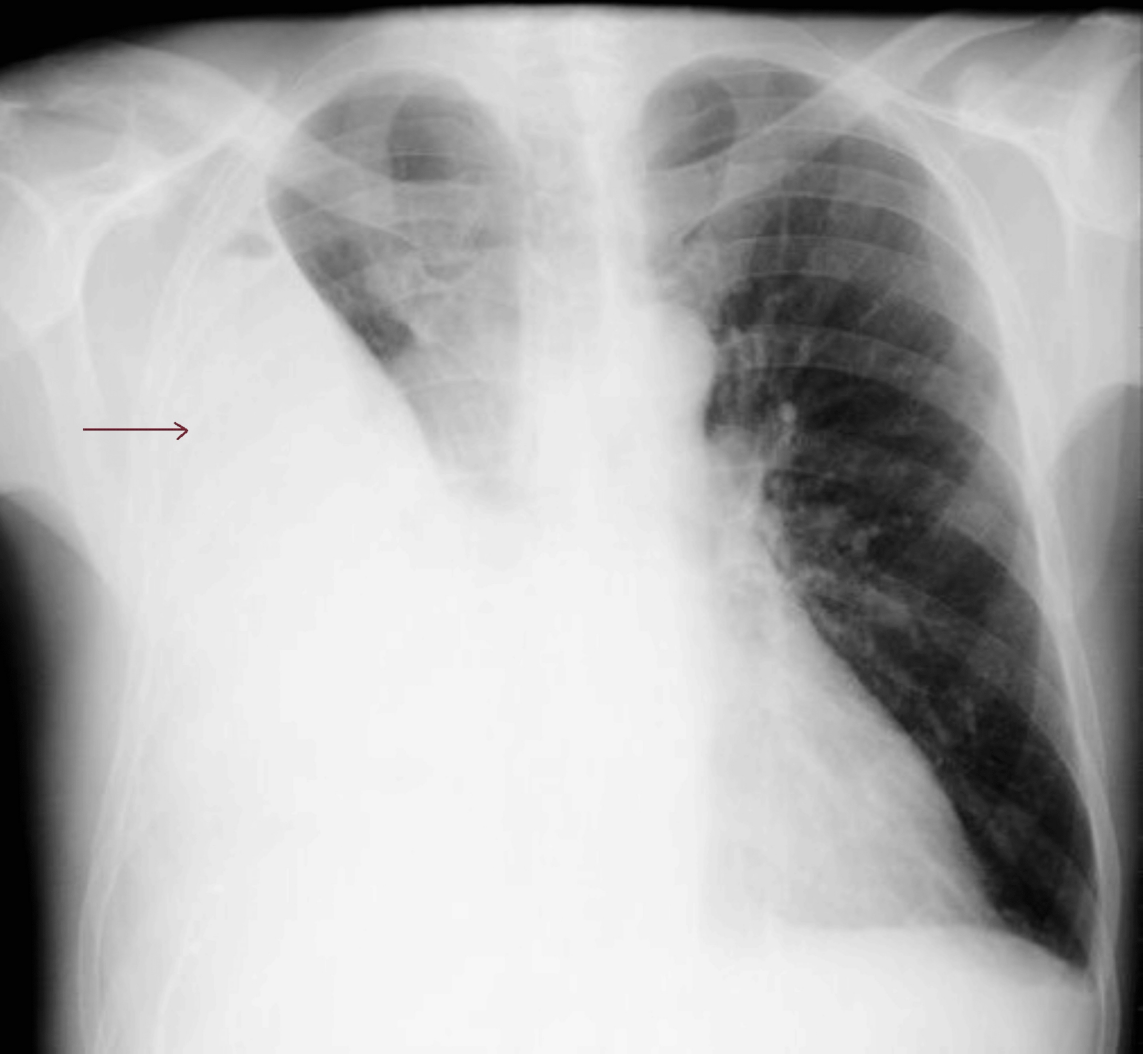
Chest X-ray performed on the third day of hospitalization shows worsening of the hypotransparency in the right hemithorax (arrow), posteroanterior view.
